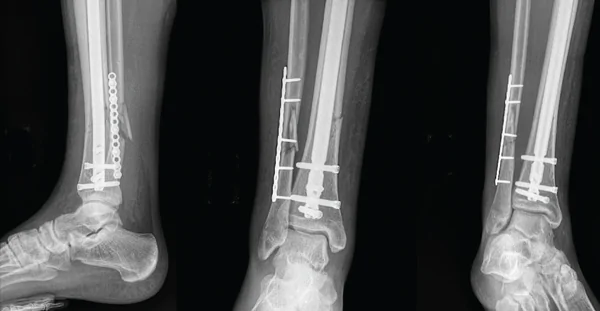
Postoperative radiographs showing intramedullary tibial nailing and fibular plate fixation.
